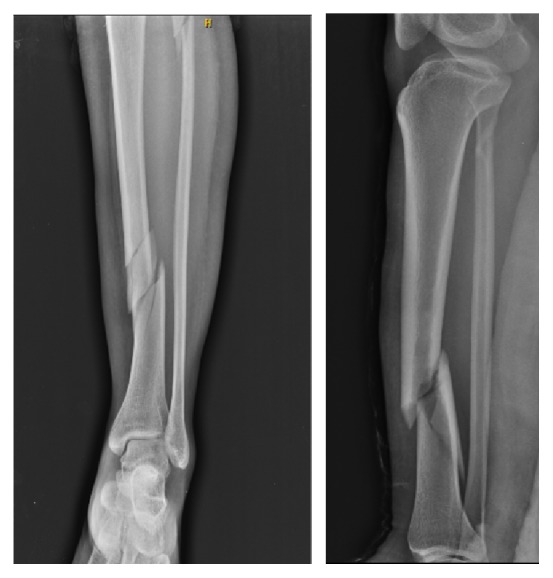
Radiographs showing the anteroposterior and lateral views of tibial shaft fracture.
